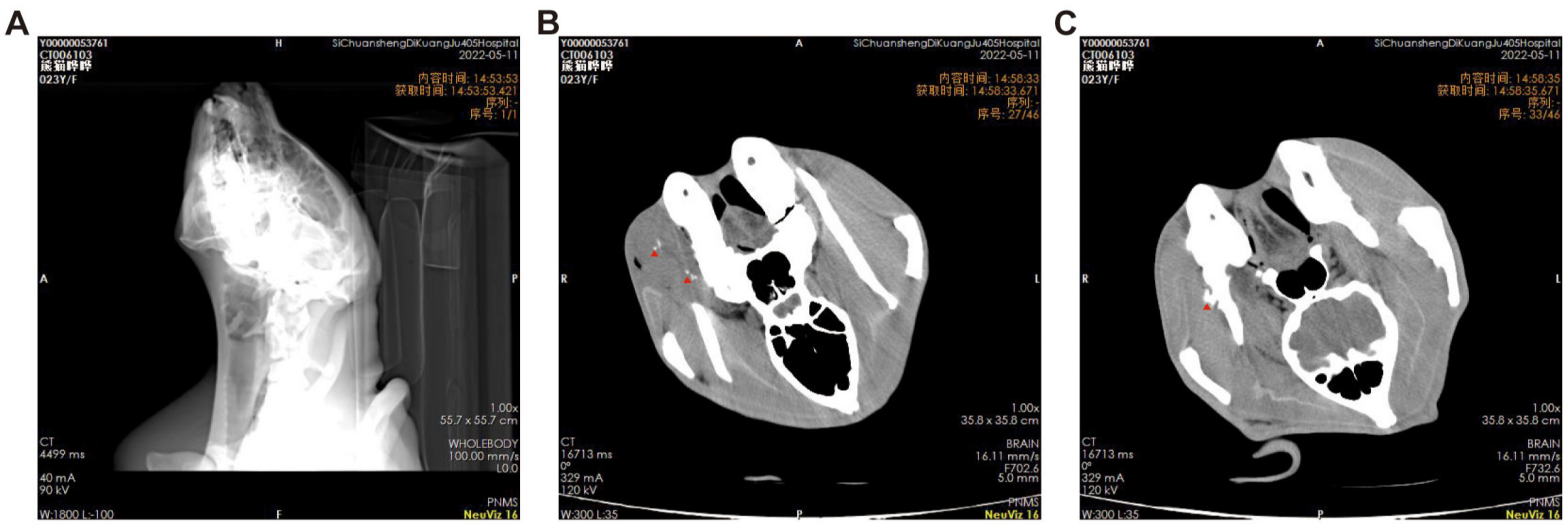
(A–C) Cross-sectional computed tomography images of the giant panda's head, the red triangle indicates the location of tumor calcification.
